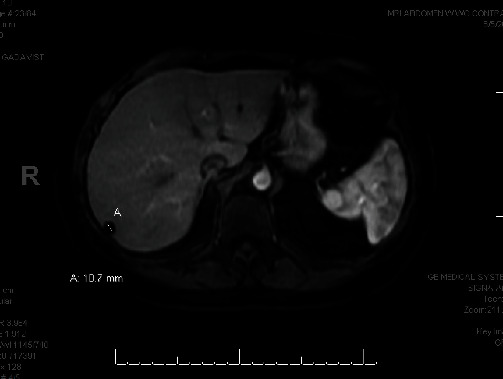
MRI of the abdomen with contrast showing benign small cysts in the right lobe of the liver.
